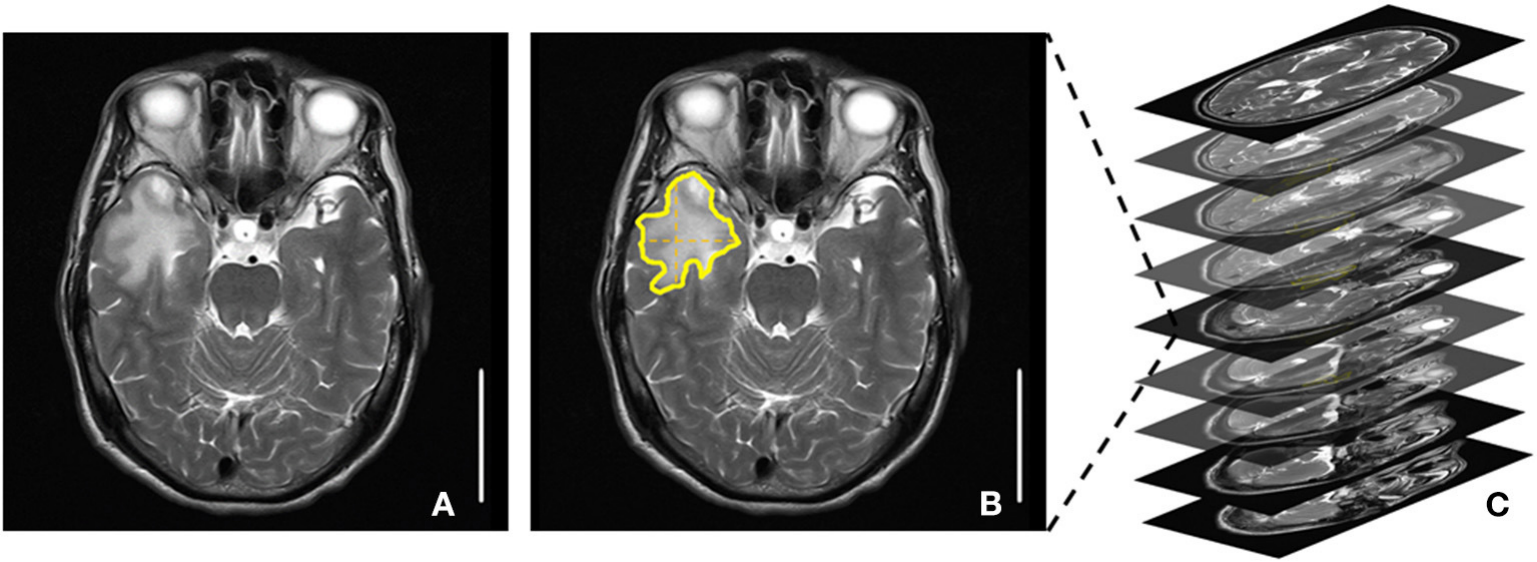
Radiation encephalopathy lesion volume measurements. (A) A T2 magnetic resonance imaging (MRI) image with the largest lesion area. (B) The largest lesion area: area surrounded by yellow lines. (C) The number of T2 MRI images with lesions. The RE lesion volume = the largest lesion area × the number of T2 MRI images with lesions.
